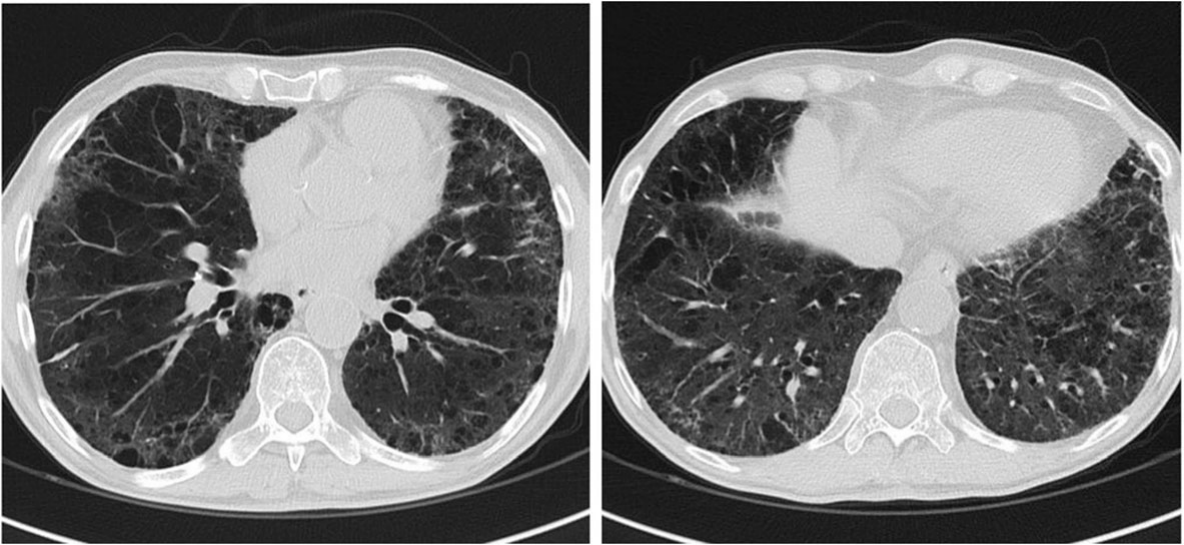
Chest CT scan image obtained 1 month after the start of imatinib mesylate demonstrates resolution of ground-glass opacities and parenchymal consolidation
